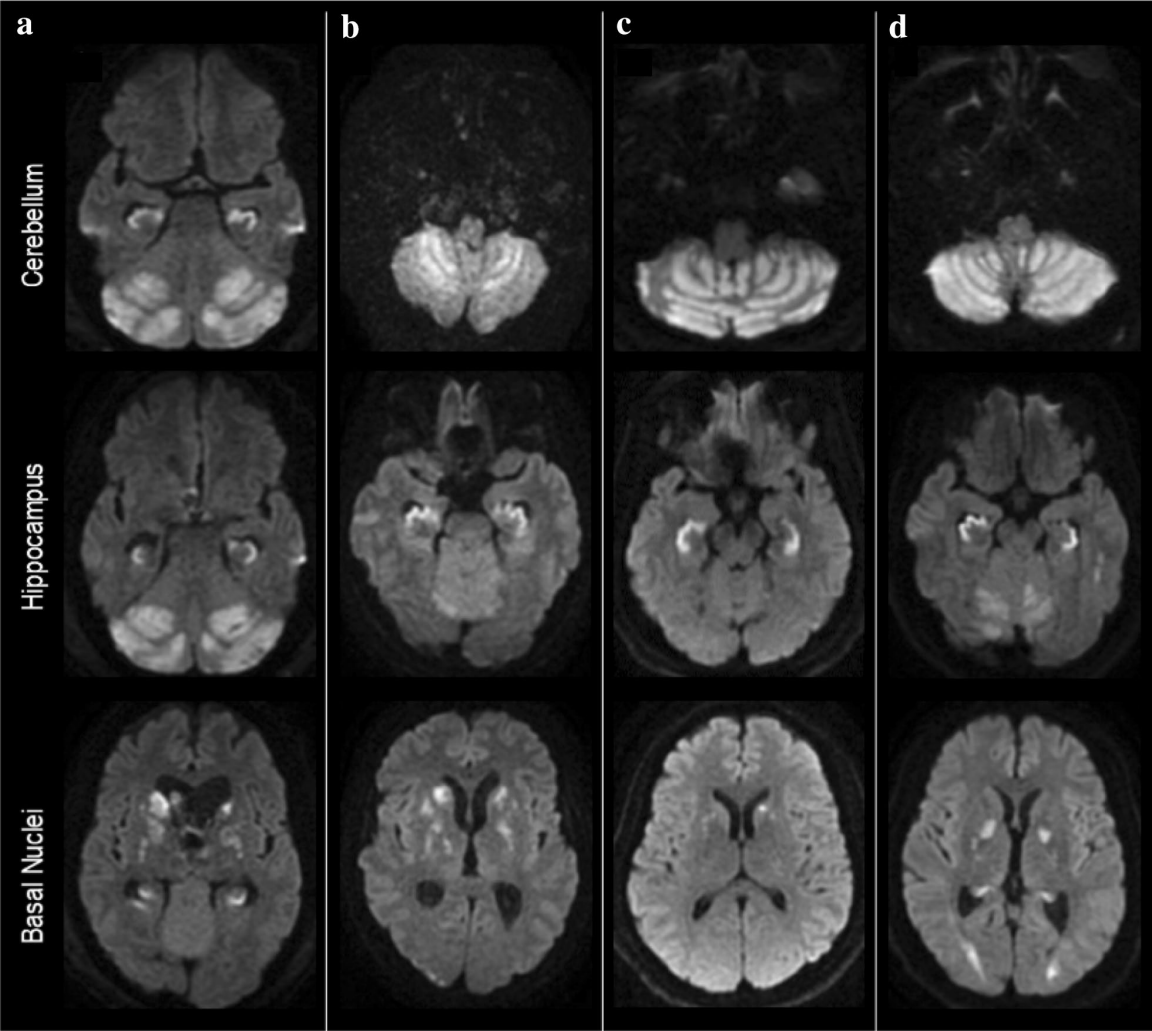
Diffusion-weighted magnetic resonance imaging by patient and brain region demonstrating cytotoxic edema in the cerebellar cortices, hippocampi, and basal ganglia of patients #1 (b), #2 (a), #4 (c), and #5 (d). MRI of patients #3 and #6 (not pictured) showed similar patterns
